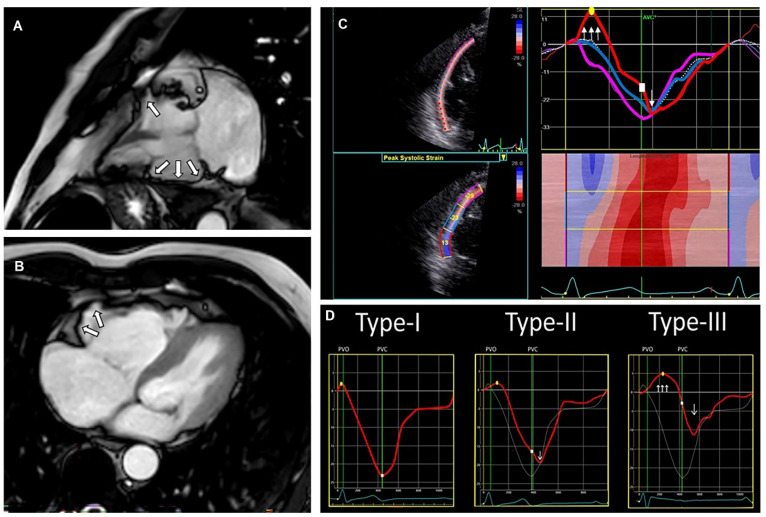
Cardiac magnetic resonance (CMR) images from a patient with arrhythmogenic cardiomyopathy (ACM), including a typical echocardiographic deformation imaging pattern. CMR images (A,B) with right ventricular (RV) aneurysm (white arrows). Echocardiographic deformation imaging (C) with RV focused and peak systolic strain measurements and deformation pattern. Distinct types of RV deformation patterns (D) as determined by Mast et al. [47]; type-I: normal pattern; type-II: “delated onset of shortening and decreased systolic peak strain”; type-III: “systolic stretching and passive recoil or shortening during early diastole”. The patients’ strain pattern shows a type-III strain pattern, fitting structural ACM.
